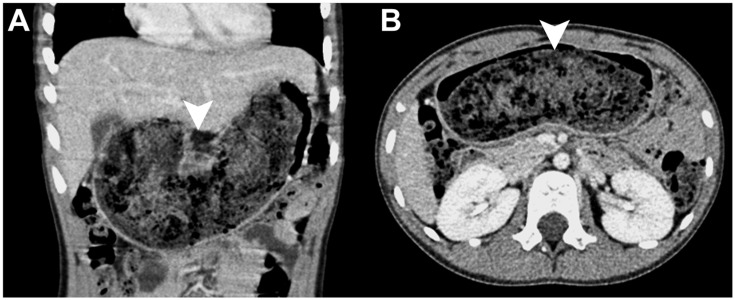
Contrast-enhanced computed tomography of the gastric trichobezoar. The contrast-enhanced abdominal CT demonstrates a large intragastric lesion with a heterogeneous, air-mixed density (arrowheads), consistent with bezoar formation, shown on coronal (A) and axial (B) images. Previous reports describe gastric trichobezoars on CT as well-defined intraluminal masses with a characteristic mottled appearance caused by numerous entrapped air bubbles within the tightly woven hairs. The lesion typically fills the gastric body and fundus and may extend beyond the pylorus into the duodenum or small bowel in Rapunzel syndrome or appear as separate small bowel masses [2,3,5,6]. Accordingly, abdominal CT is considered the single most effective modality for staging trichobezoars, enabling the assessment of the lesion extent, small bowel involvement, subsidiary bezoars, and obstruction or ischemic risk; evaluations of all are essential for surgical planning [3,7].
